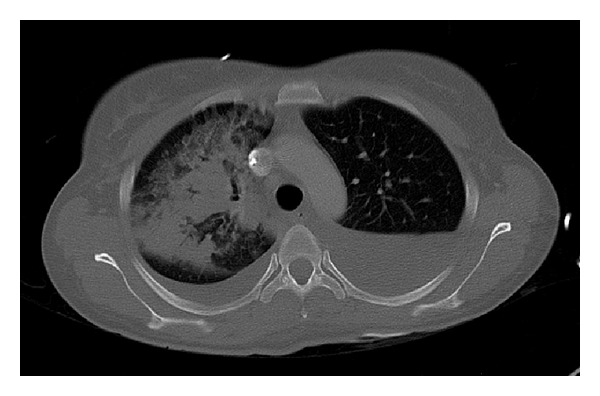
CT-scan showing* de novo* consolidation of the right lung, 24 hours after surgery.
